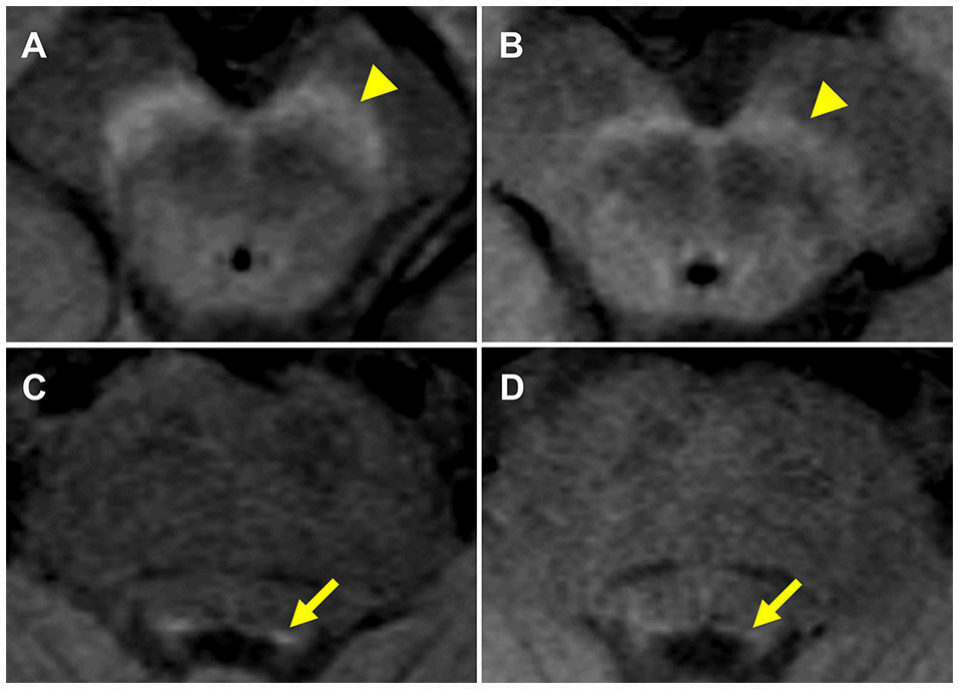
NM-MRI of the substantia nigra and locus coeruleus. NM-MRI images at the levels of the substantia nigra (arrowhead) and the locus coeruleus (arrow) of a 66-year-old healthy woman (A,C) and a 70-year-old man with PD (B,D).
